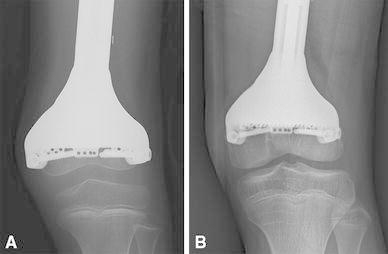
a An anteroposterior (AP) radiograph of the left knee shows a joint-preserving tumor resection and custom expandable prosthetic reconstruction in an 8-year-old boy. b An AP radiograph of the left knee taken 6 years after surgery shows continuous growth of the remaining distal femur epiphysis. More conservative resection and accurate reconstruction may be performed with the CATS technique, because less soft tissue dissection is required to define the intended resection planes intraoperatively and the blood supply to the remaining epiphysis is preserved
